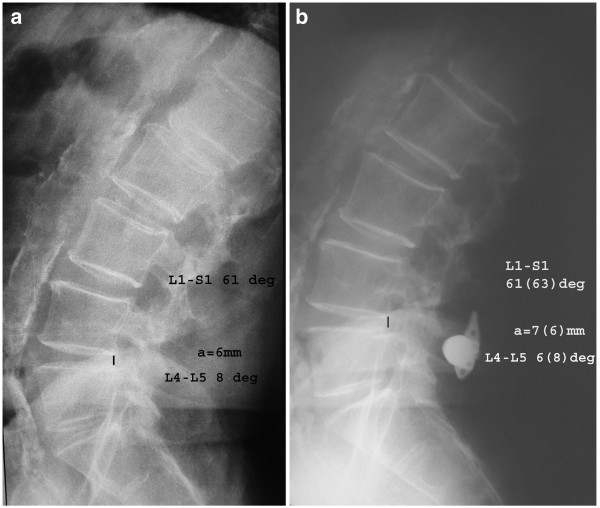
Preoperative (A) and postoperative (B) lateral lumbar radiograph of a patient treated with x-stop insertion and left direct lateral decompression showing increase of posterior intervertebral disc height and preservation of lumbar lordosis.
